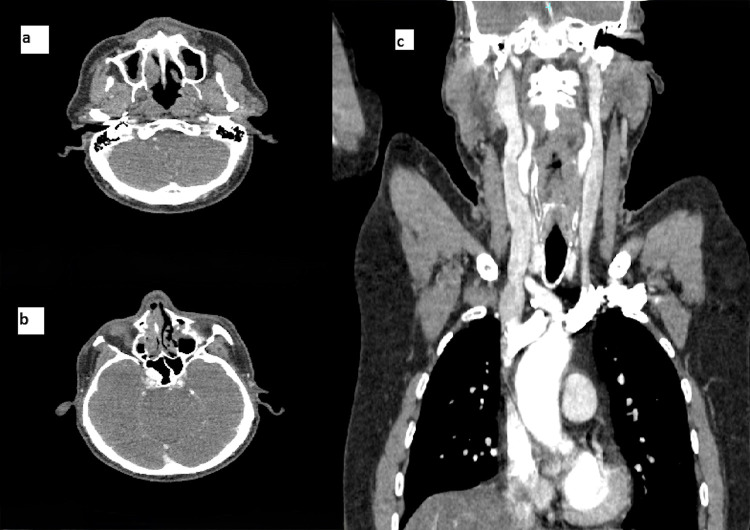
A follow-up contrast-enhanced CT scan. (a) Axial section showing the absence of air specks in the upper neck in the retrodental region and pterygoids. (b) Axial section showing normal enhancement of cavernous sinuses with no air specks. (c) Coronal contrasted neck CT showing patent internal jugular veins and normal vasculature with no sir specks in the soft tissues.
